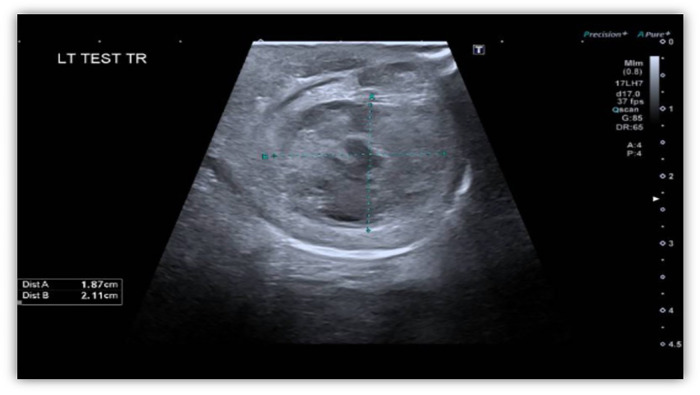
Doppler ultrasound of left testis: a single transverse grayscale sonographic image of the left testicle demonstrates a heterogeneous, predominantly echogenic lesion within the left testicular parenchyma. The echogenic lesion measures 1.9 cm × 2.1 cm × 2.5 cm. The differential diagnosis for the echogenic lesion includes hematocele, hematoma, abscess, or neoplasm.
